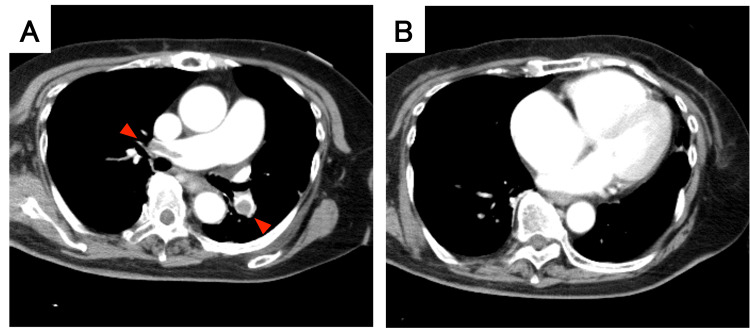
CT pulmonary angiography CT showing pulmonary embolism. (A) Emboli are observed in the bilateral pulmonary arteries (red arrows). (B) Severe right ventricular enlargement is evident. No segmental left ventricular perfusion defects were observed. CT: computed tomography
